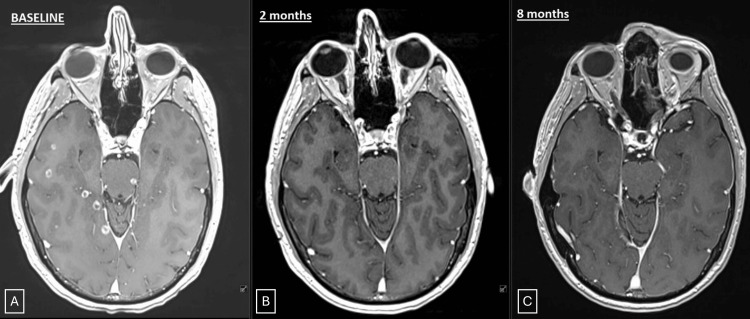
Serial contrast-enhanced MRI images. A: Axial sections of baseline contrast-enhanced MRI scan demonstrating multiple metastases, including brainstem lesions. B: Follow-up scan at two months. C: Follow-up scan at eight months.
